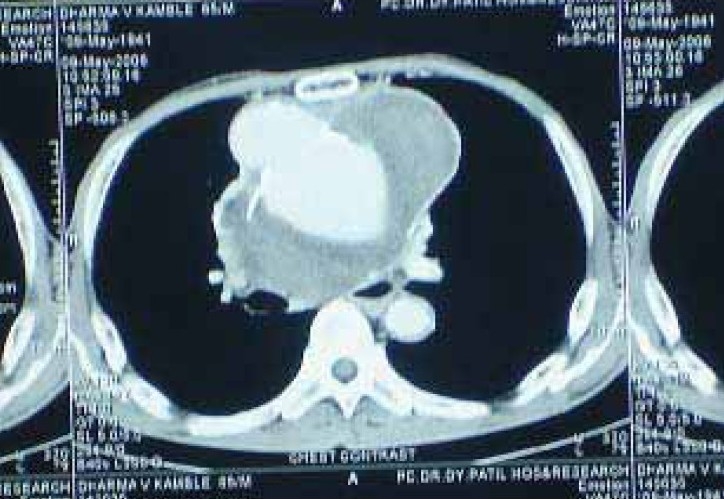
CT Thorax showing aneurysm abutting sternum and vertebrae, calcification of aortic wall & thrombus in aorta
